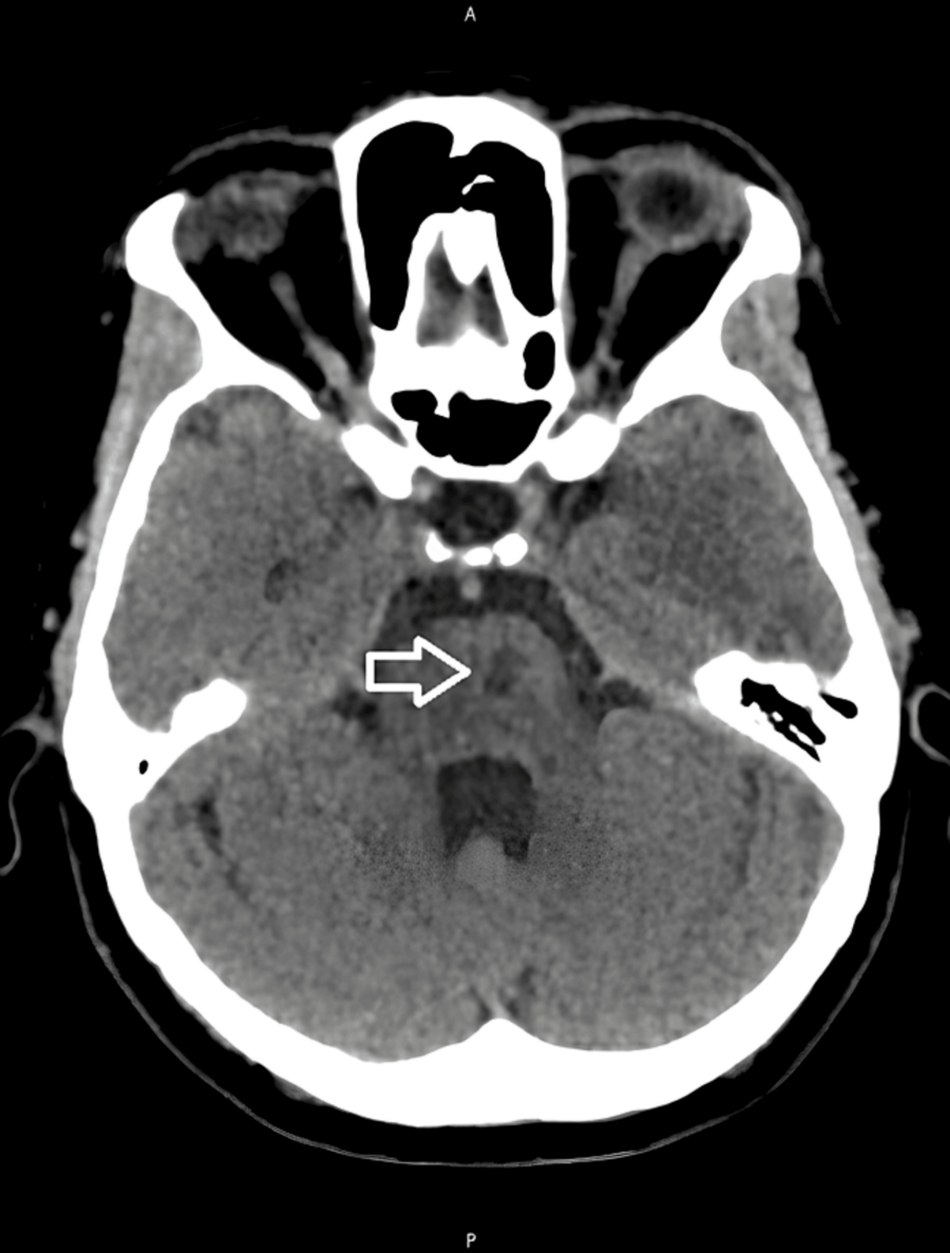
A plain CT head showing a left paramedian pons hypodensity suggestive of an infarct of indeterminant age.
